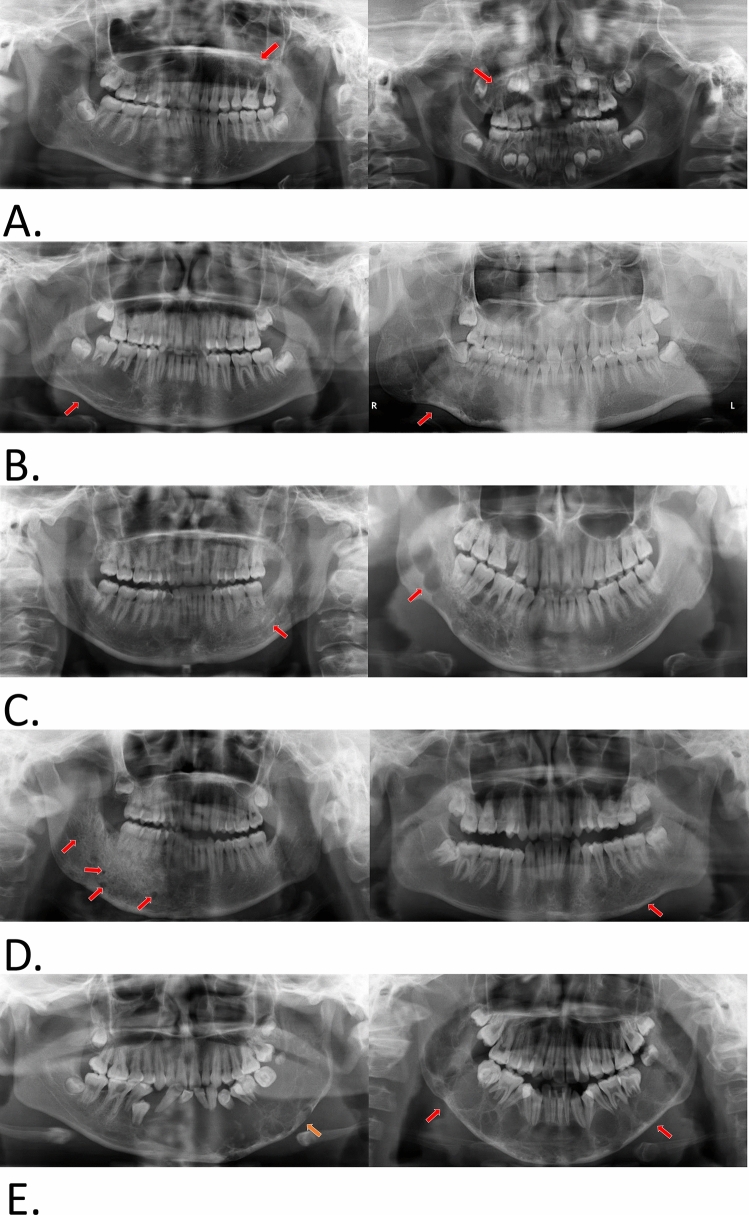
Orthopantomogram manifestations of j-AVMs in the maxilla (a) and mandible (b–e). (a) Unclear maxillary sinus and mild ground-glass appearance with involvement of the region around the roots of incisor (left) and first molar (right). The arrow indicates unclear maxillary sinus. (b) A clear oval or irregular hypodense shadow is mostly centred on the root of the first molar with a long axis along the mandibular canal and occasionally with root resorption. (c) Enlarged and wider mandibular canal than that on the contralateral side, and the mandibular canal was replaced by an irregular hypodense shadow. (d) Ground-glass appearance with an unclear edge and single or multiple “soap bubble” lucencies (indicated by the yellow arrow). (e) Osteolytic and polycystic changes involving a large part of the mandible with signs of teeth root displacement and enlargement of the involved region.
